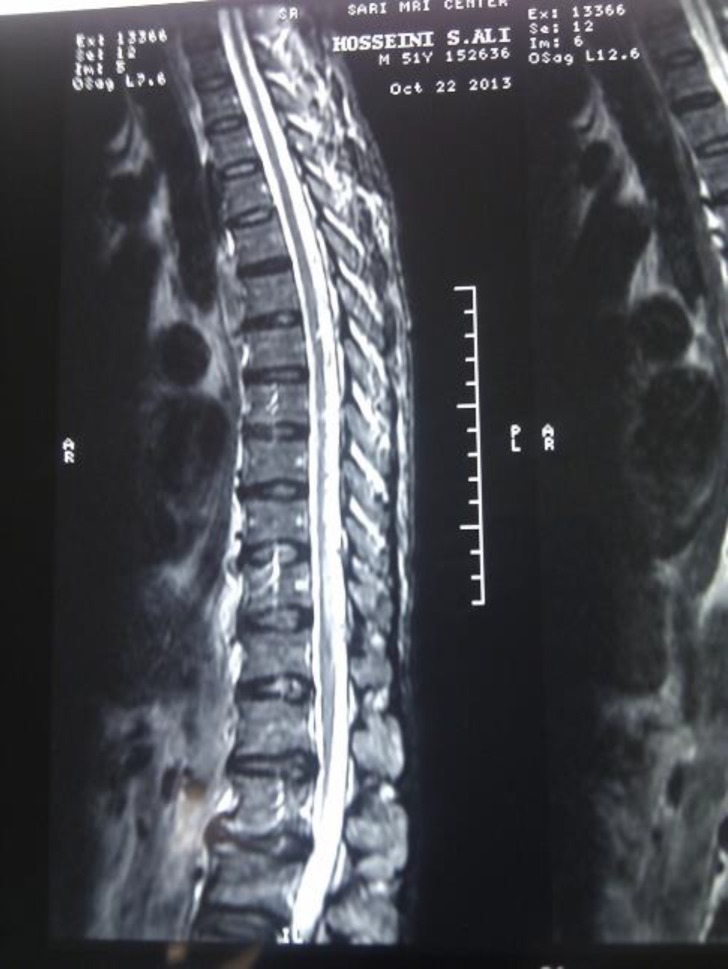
Sagittal T_2_- weighted magnetic resonance image of the thoracolumbar spine showing edema of the thoracic cord and regional dilated perimedullary vessels suggestive of a spinal vascular malformation
